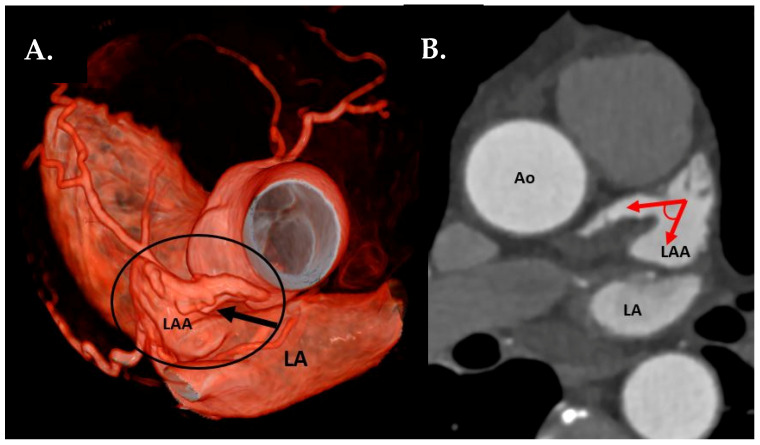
(A) CTA 3D-VRT reconstruction of LAA with a sharp tip angulation (≤90°) (black arrow). (B) sagittal plane with red arrows delineating sharp LAA tip angulation. Ao—aorta, LA—left atrium, LAA—left atrial appendage.
